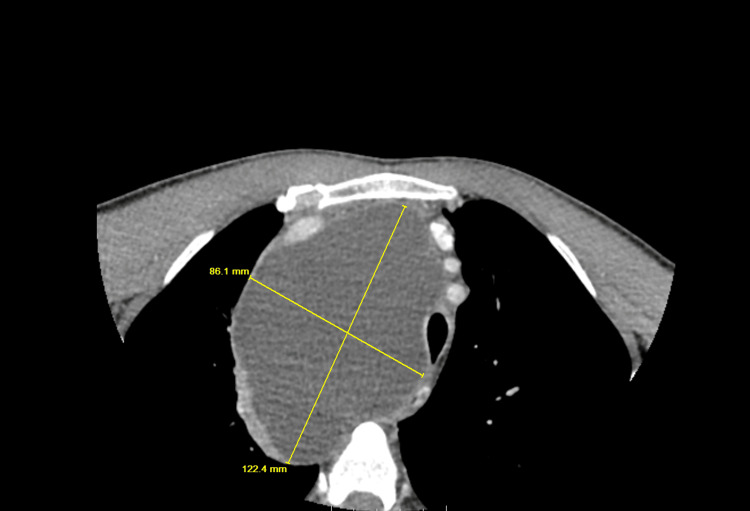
CT neck and chest showing a thyroid mass with mediastinal extension and mass effect CT of the neck and chest demonstrates a large cystic mediastinal extension measuring up to 12.2 cm, likely representing a cystic component of the thyroid mass, with a significant mass effect causing displacement of the larynx, trachea, and esophagus. CT, computed tomography.
